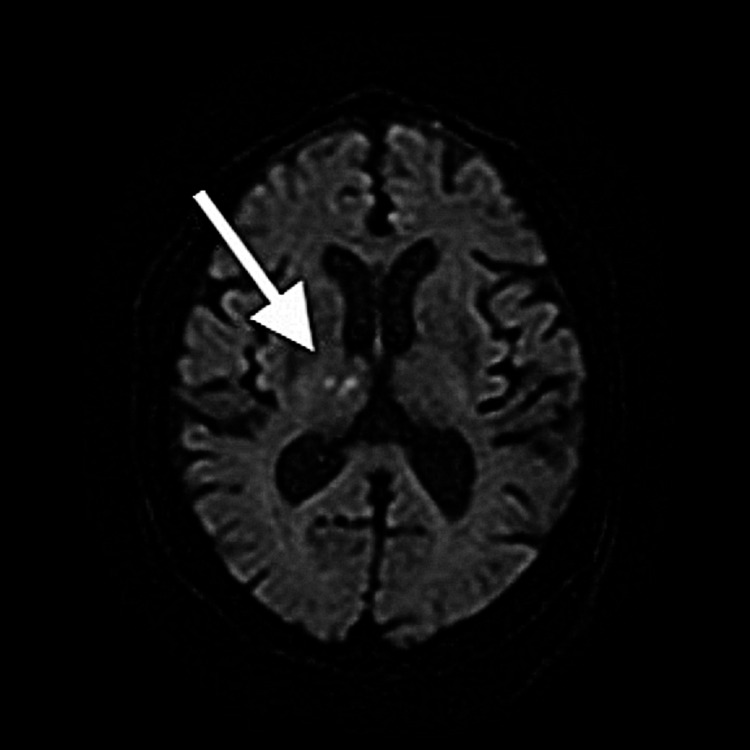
Brain MRI image Brain MRI on six days from admission in Case 2 shows infarctions in the right thalamus
